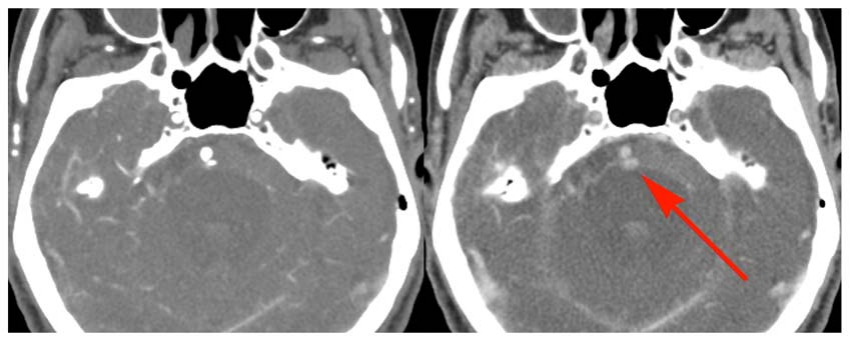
Example of active bleeding from a ruptured aneurysm. 86 year old male with two day history of lethargy with progressive stroke like symptoms. Early and delayed phase CTA images show subarachnoid hemorrhage with a 2(red arrow), consistent with active bleeding. Because of the patient's poor prognosis, and in adherence with his wishes, no intervention was performed. The presence of active bleeding on the delayed views did not change this management decision.
